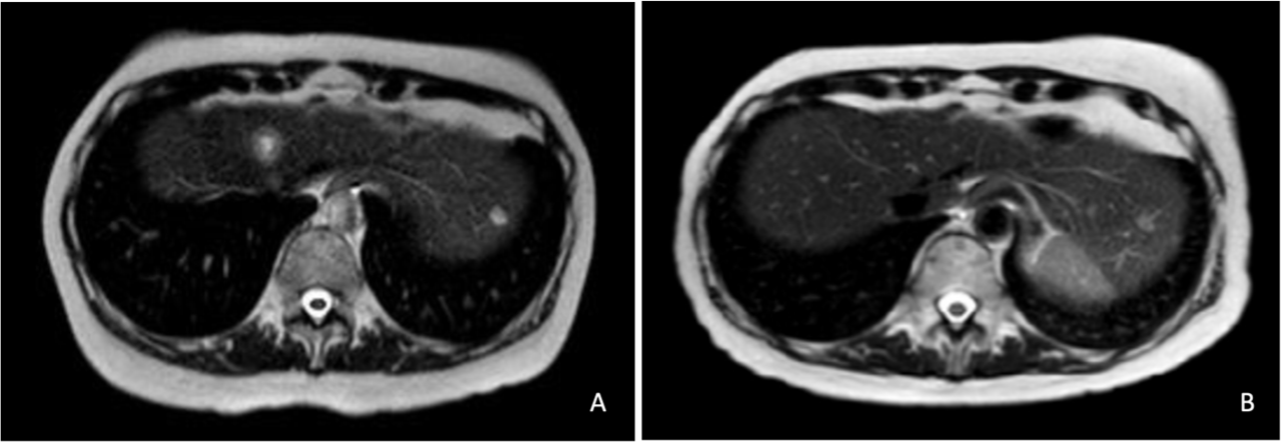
MRI study showing two liver metastases in January 2017 (A). Complete response of liver lesions after 2 cycles of talazoparib in April 2017 (B).
